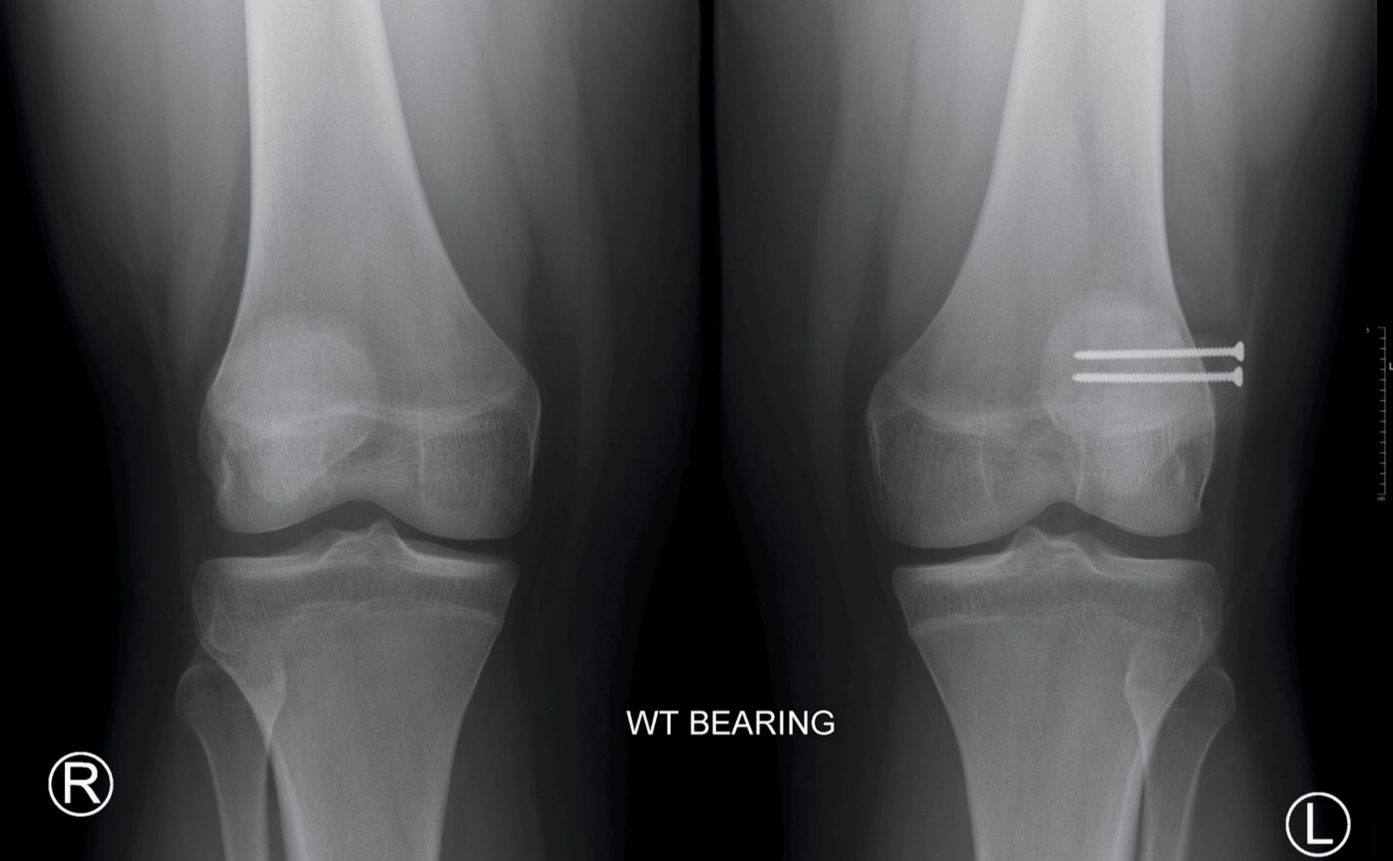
12-month postoperative X-ray B/L knee AP view AP: Anteroposterior; B/L: Bilateral.
